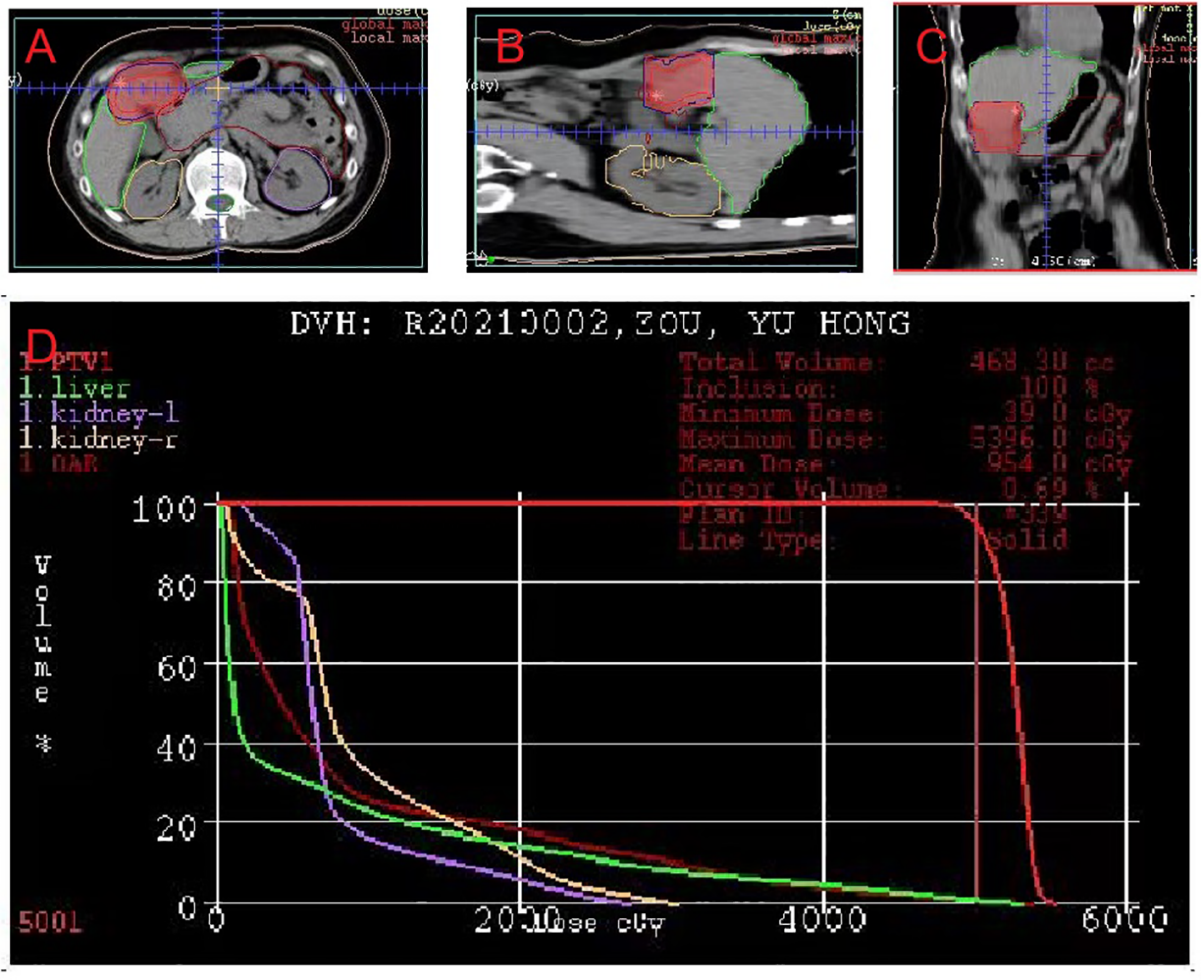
Dose-planning of radiotherapy. Planned target volume (GTV: red, PTV1: blue) covered by the 95% isodose can be seen in (A) axial, (B) coronal, and (C) sagittal planes on pretreatment planning computed tomography. (D) Dose-volume histogram shows that 95% of the PTV volumes irradiated with more than 5000 cGy.
